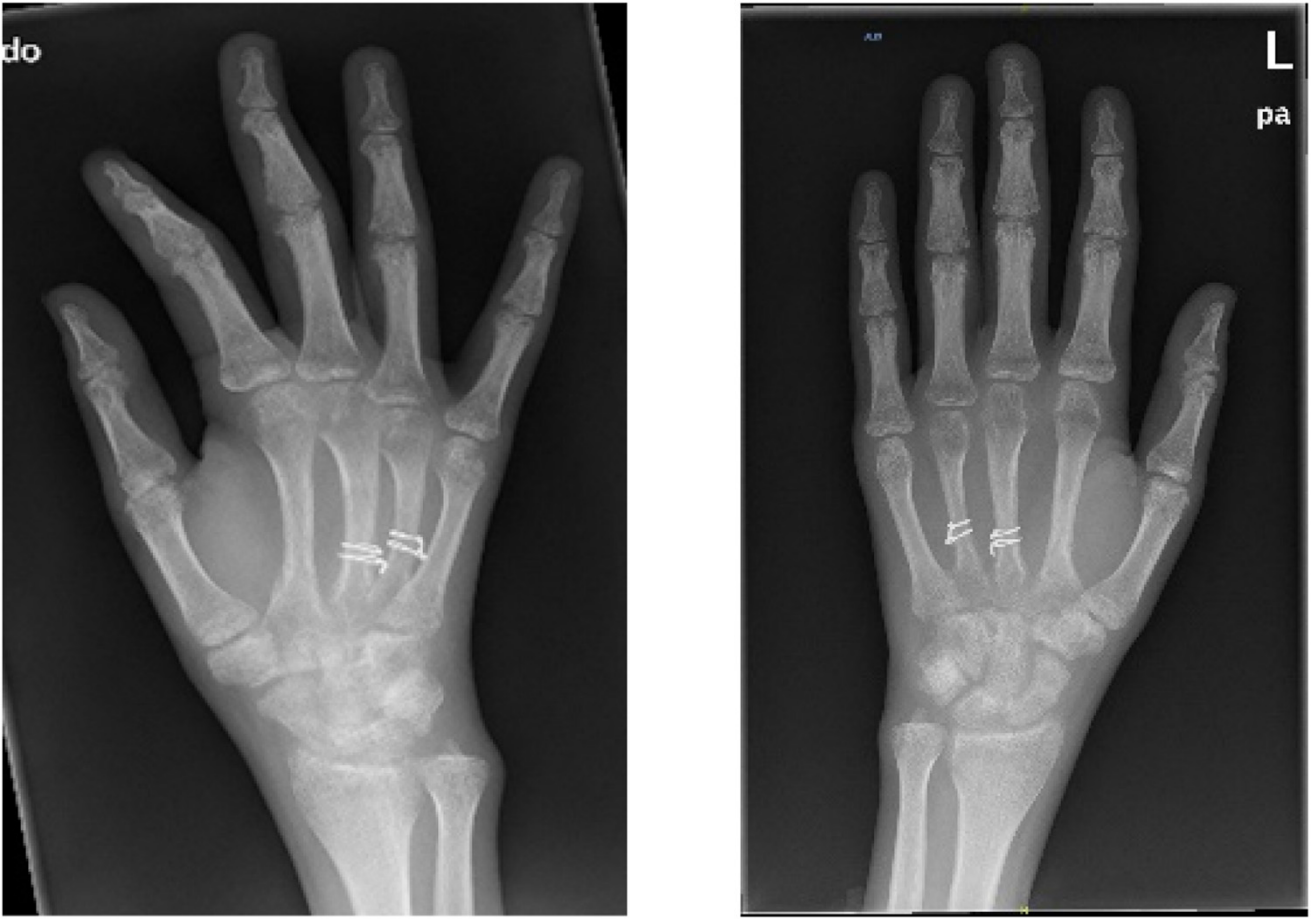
Post-operative X-ray of the middle and ring metacarpal shaft fracture at 4 months.
